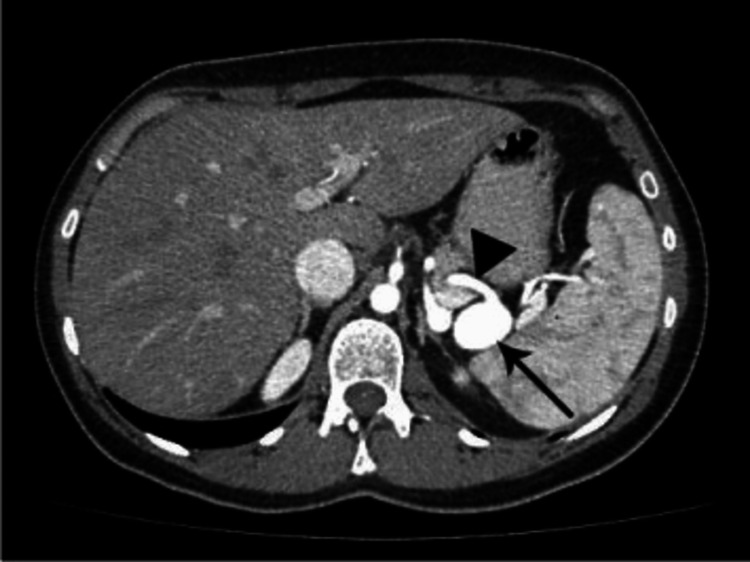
Contrast-enhanced CT scanning demonstrating the saccular aneurysm of the splenic artery (arrow).
